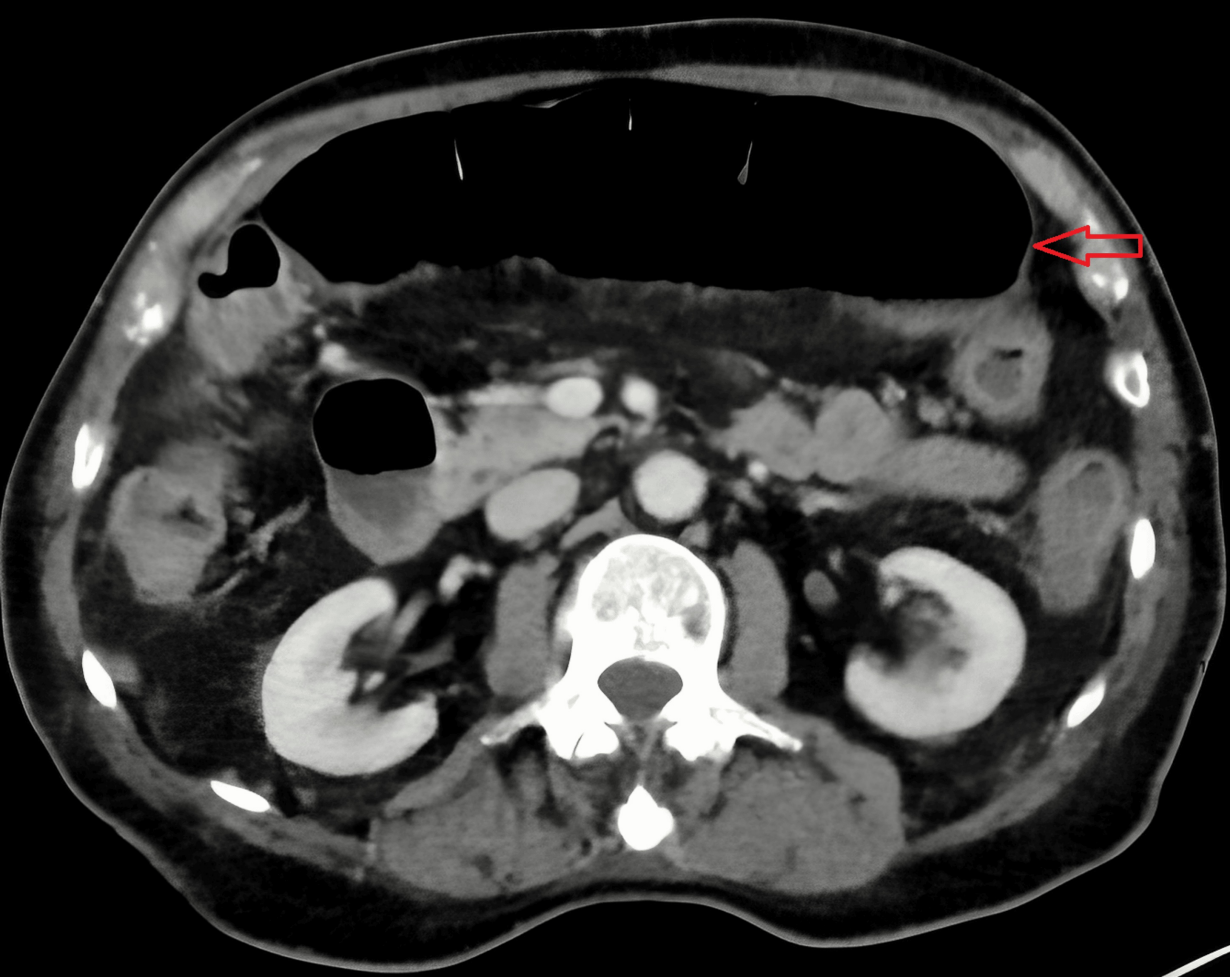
CT scan of abdomen and pelvis on day three. The transverse colon (red arrow) is dilated measuring 5.7 cm in the anteroposterior diameter and 7.7 cm in the craniocaudal diameter consistent with megacolon.
